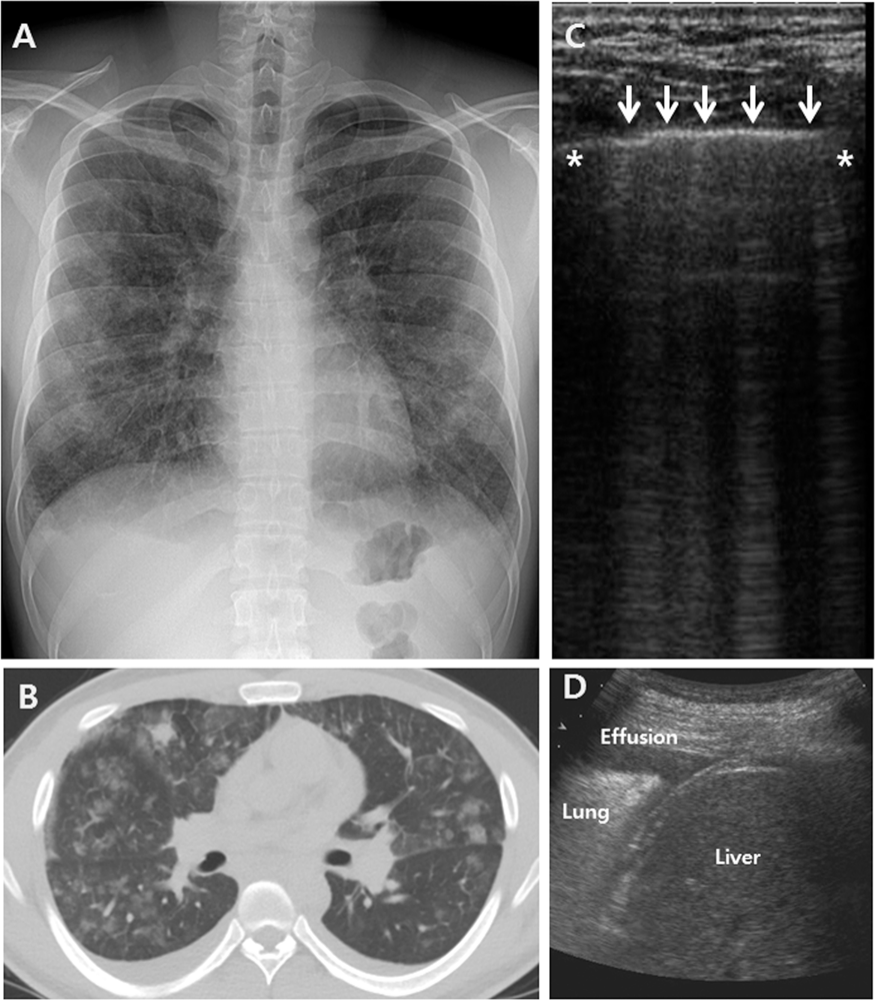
Examples of common thoracic ultrasound (TUS), radiographic, and computed tomography (CT) findings in AEP patients. (A) Chest radiographs revealed bilateral diffuse ground glass opacity (GGO) with reticular opacities and mild blunting of both costophrenic angles. (B) Chest CT images revealed bilateral diffuse GGO with interlobular septal thickening and patchy consolidations bilateral pleural effusion. (C) TUS imaging of the right upper anterior lung zones revealed discrete laser-like vertical hyperechoic reverberation artifacts that arose from the pleural line, multiple B-lines (white arrows) and pleural effusions (D) (asterisk = ribs).
